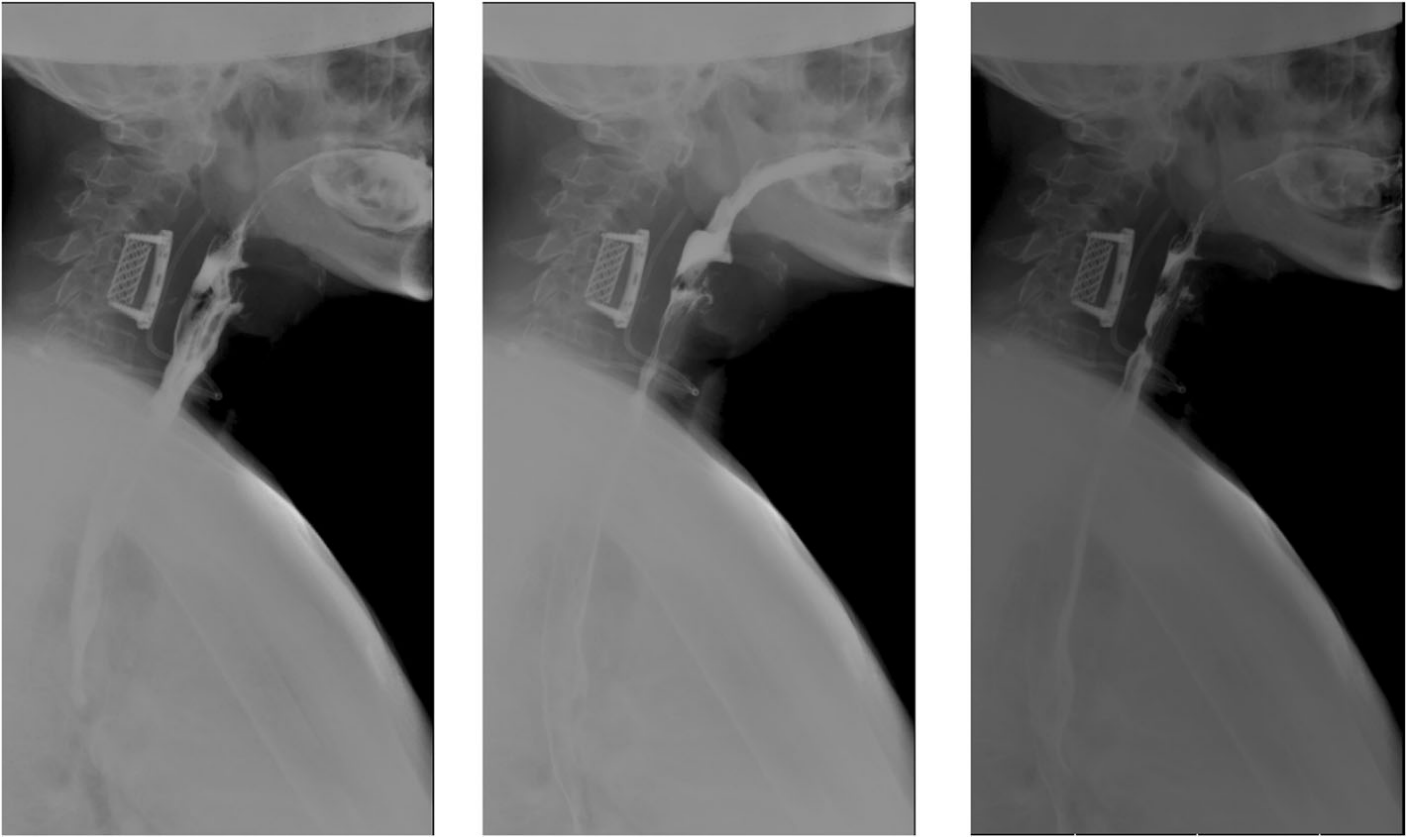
Barium-swallow radiograph
